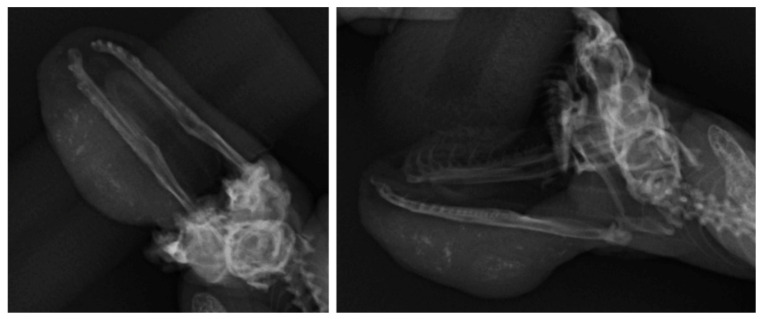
Radiographic examination of the submandibular mass revealed the presence of multi-focal mineralization in the area of the lesion. No evidence of mandibular invasion and lung metastasis was observed.
